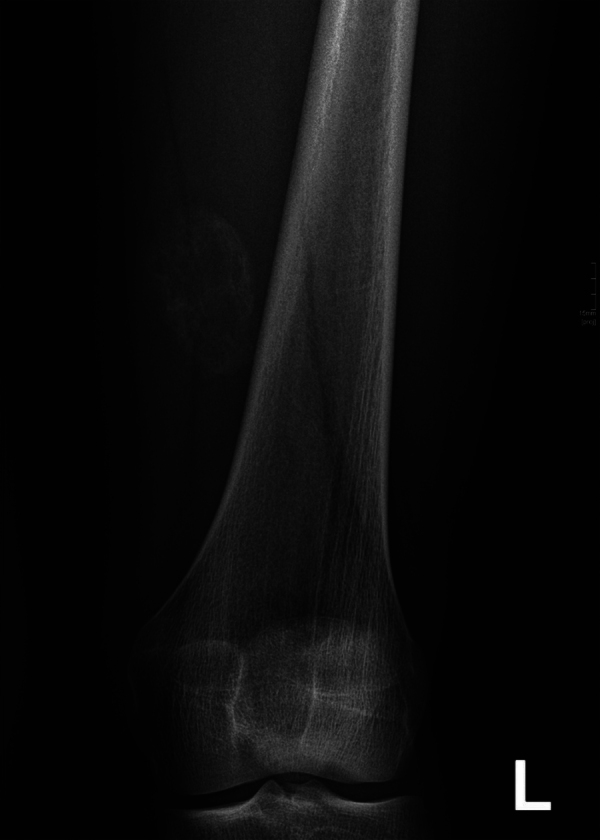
Radiograph of myositis ossificans shows progressive, oval, intramuscular ossification.
